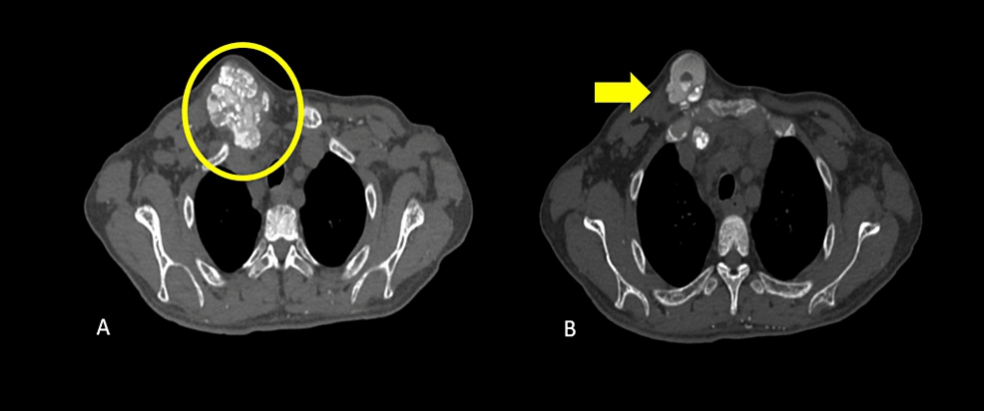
Plain CT of the chest Axial (A, B) views showing multilobulated, calcified lesions of the right sternoclavicular joint (circles), with a few demonstrating sedimentation (arrow). The mass measures 4.5x6.2x6 cm (CCWAP). CT: computed tomography
